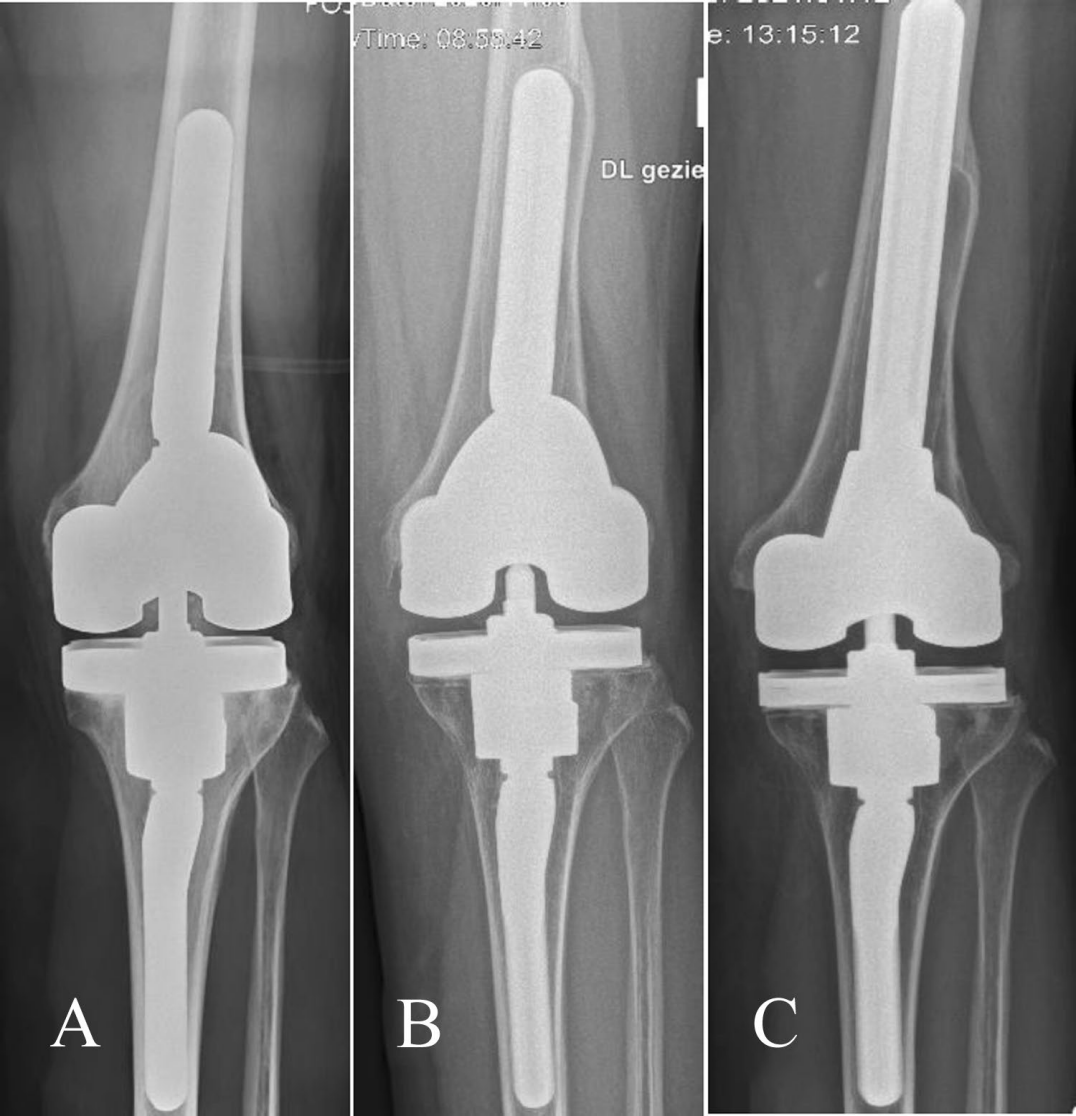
A Left knee, anteroposterior radiographs show perfect implantation of a condylar constrained knee (CCK) component with cementless stems and TMC on tibia only. B Left knee, anteroposterior radiographs show loose femur component with stable tibia construct for aseptic loosening after 5-year FU. (C) Left knee, anteroposterior radiographs show partial revision of femur component only with TMC using a longer cementless stem
